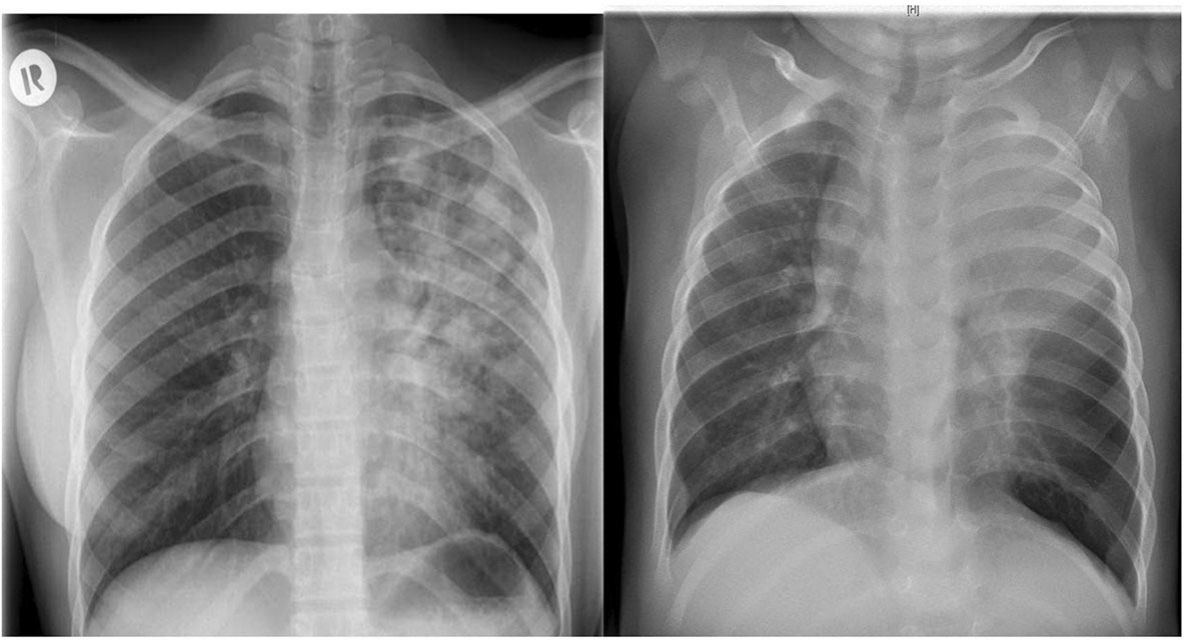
Chest X-ray studies showing extensive TB-lung involvement in both the adult (left) and the pediatric patient (right)
